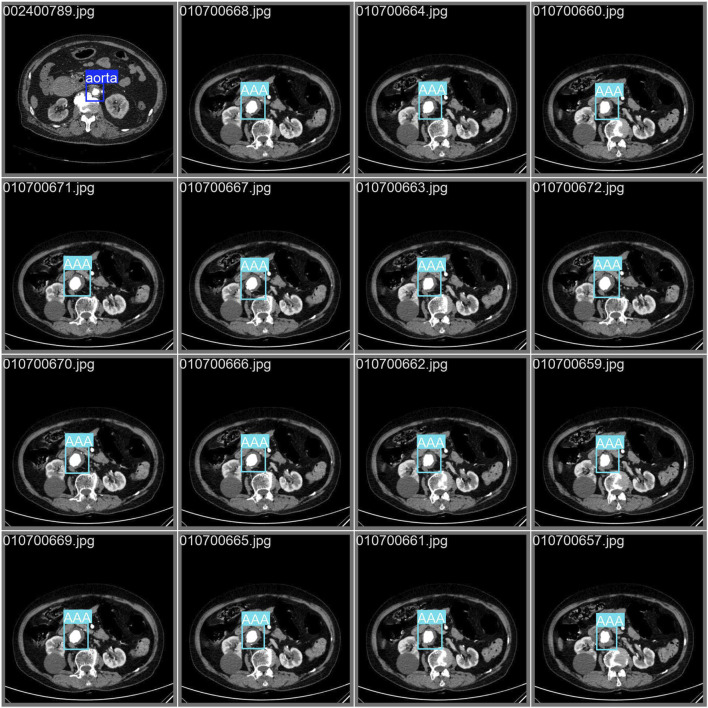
Validation for classifying the normal aorta and abdominal aortic aneurysm of the YOLOv11 model on CTA images. YOLO, You Only Look Once; CTA, computed tomography angiography.
